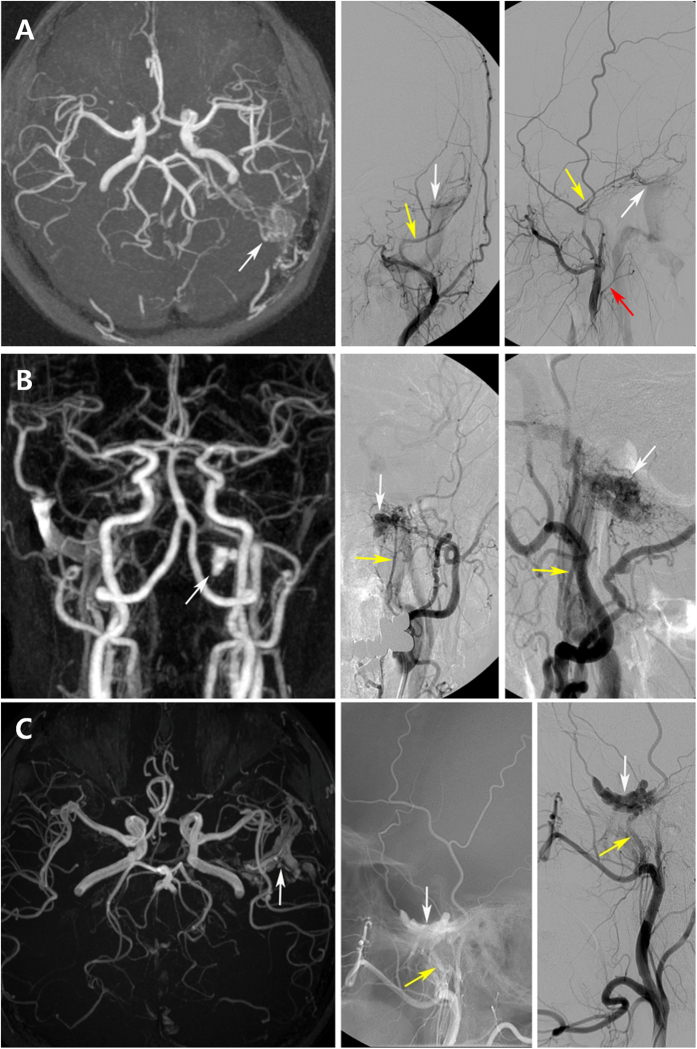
Representative brain MRI images and TFCA findings of (A) transverse-sigmoid sinus dAVF, (B) hypoglossal canal dAVF, and (C) middle cranial fossa dAVF. (A) Left external carotid artery angiogram in anteroposterior and lateral views shows a dAVF with feeders from left middle meningeal artery (yellow arrows) and left occipital artery (red arrow), draining into left sigmoid sinus (white arrows). (B) Left external carotid artery angiogram in anteroposterior and lateral views shows a dAVF with feeders from left ascending pharyngeal artery (yellow arrows), draining into left internal jugular vein (white arrows at venous sac). (C) Unsubtracted and subtracted left external carotid artery angiograms in lateral view show a dAVF in left middle cranial fossa with feeders from left middle meningeal artery (yellow arrows), draining into left inferior petrosal sinus (white arrows at venous sac).
